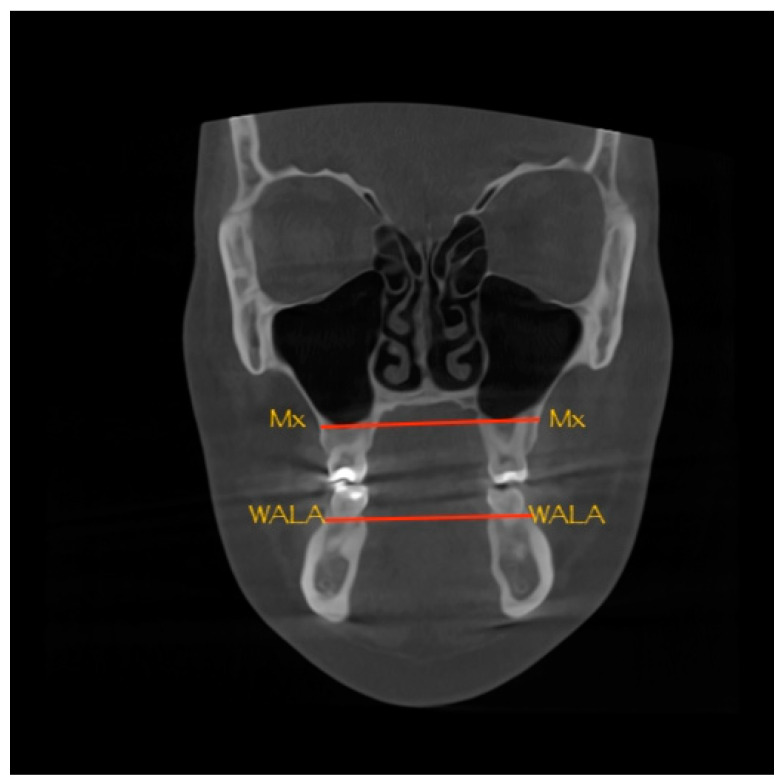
UPCBCT analysis: Mx-Mx and WALA-WALA distances.
